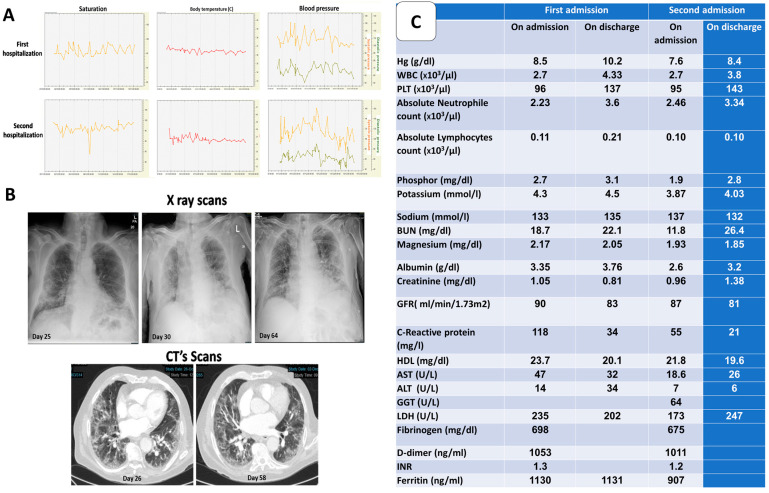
(A) O_2_ saturation, body temperature, systolic and diastolic blood pressure. The upper and lower panels relate to the first and the second hospitalization, respectively. (B) Upper panel: Posterior anterior chest radiograph showing bilateral patchy lung consolidation in a peripheral distribution pattern on days 25, 30 and 64 after the first positive corona test. Lower panel: Unenhanced, thin-section CT performed on day 26 after the first positive corona test showing bilateral ground–glass attenuations with subsequent deterioration in the second hospitalization on day 58. (C) Complete blood count and bio-chemical results of the patient during the first and the second hospitalizations upon admittance and discharge.
